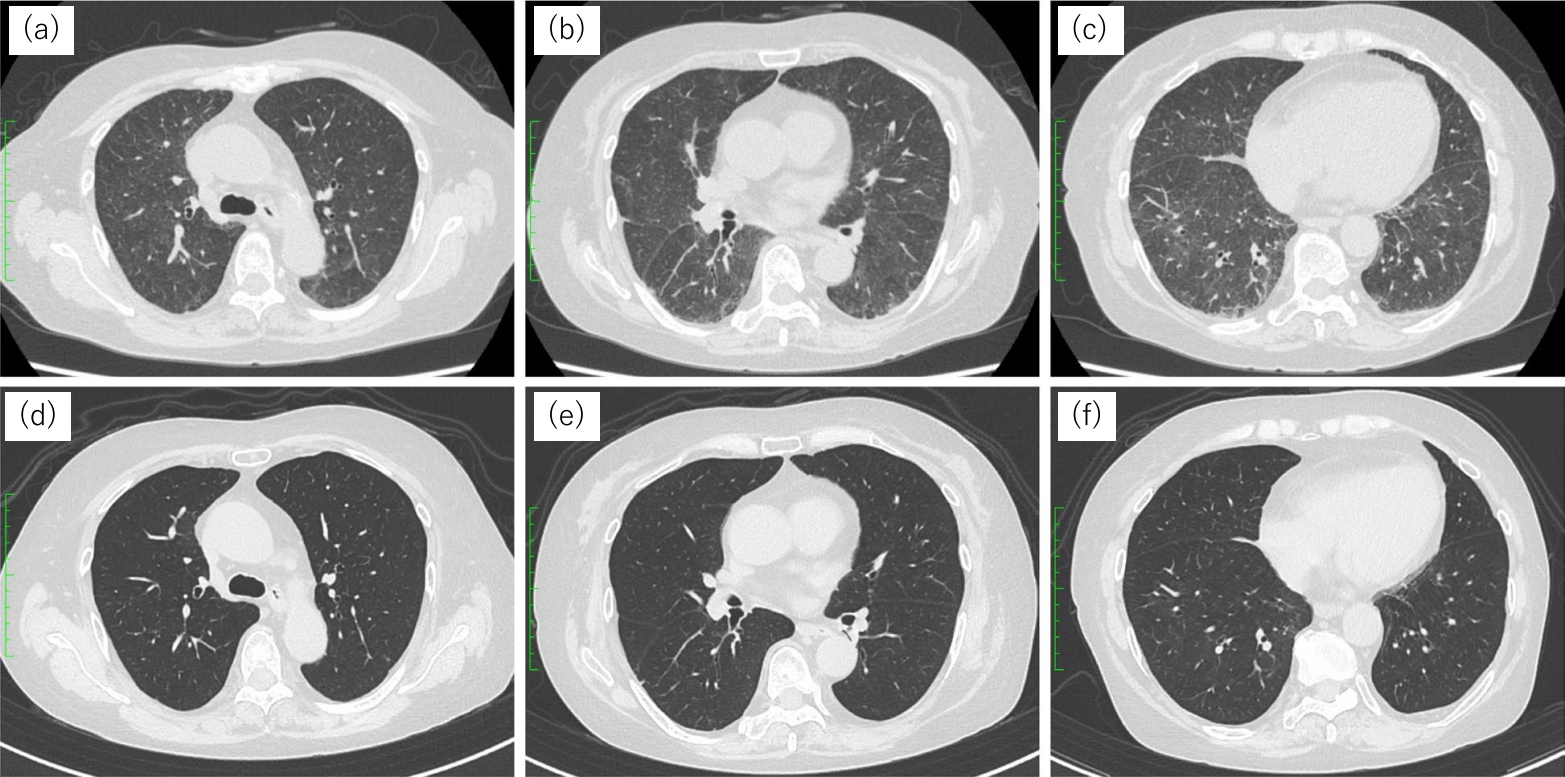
High-resolution computed tomography of the chest at the time of diagnosis in case 1, showing diffuse faint ground-glass opacity with mid- and lower-lobe predominance in both lungs (a-c). One month later, complete resolution of the shadows is observed (d-f)
